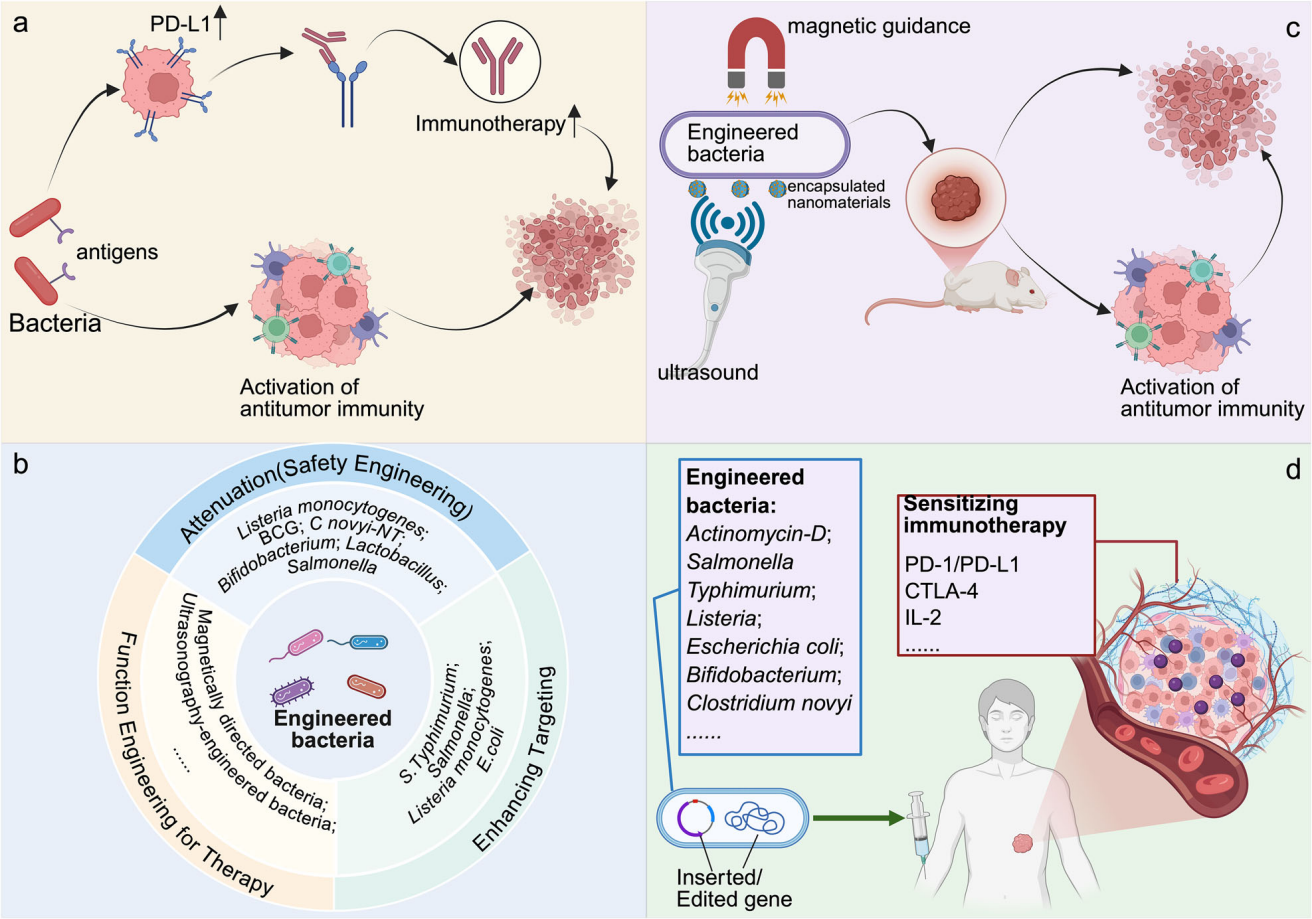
Clinical translation strategies for tumor-inhabiting microbiota-based therapeutics. a Immunotherapeutic sensitization. Intratumoral bacteria can be used as an antigenic source to improve the efficacy of immunotherapy by increasing PD-L1 expression in tumor cells and activating the antitumor immune response, thus effectively killing tumor cells. b Engineering bacteria. By modifying the natural intratumoral microbiota for safety and toxicity reduction, enhancing targeting and specific functions (e.g., drug delivery), therapeutic engineered bacteria can be constructed. c Targeted drug delivery. Engineered bacteria capable of magnetic or ultrasonic targeting may be utilized as targeted carriers to deliver nanodrugs to tumor locations, activate antitumor immunity, and kill tumor cells. d Clinical translation progress. Engineered bacteria developed on the basis of the above strategies are undergoing investigation in clinical trials for sensitizing solid tumors to immunotherapy (e.g., therapies targeting PD-1/PD-L1, CTLA-4, and IL-2). Created with BioRender.com.
